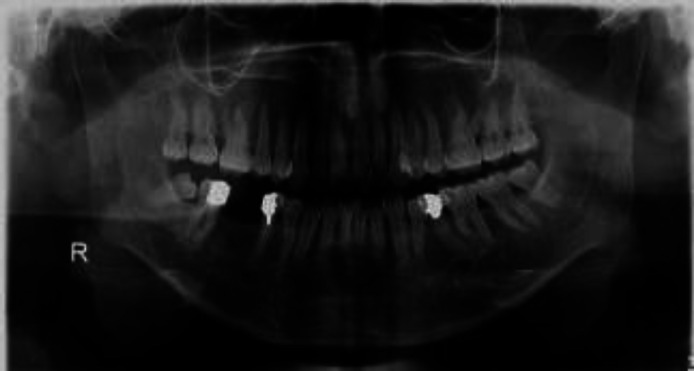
Panoramic radiography showing obliteration of maxillary sinus in thalassemia major
